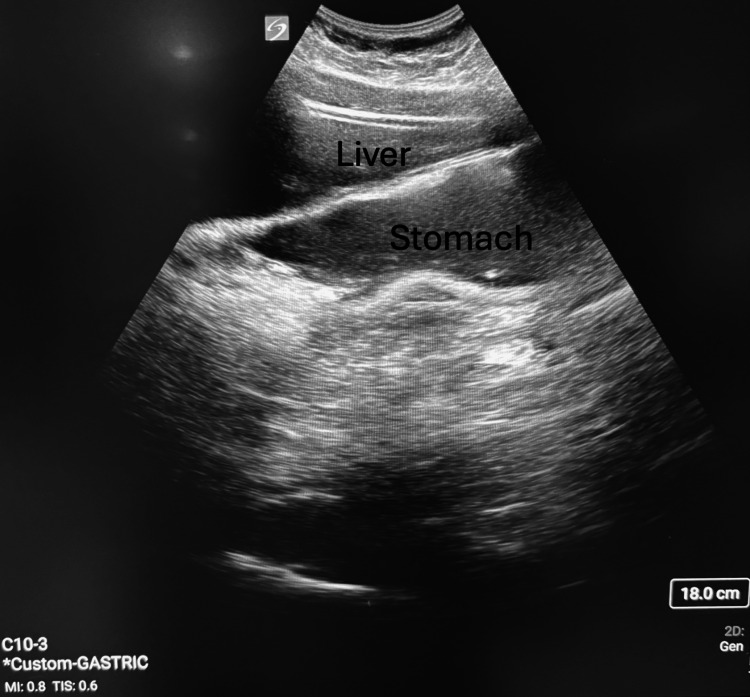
Preoperative gastric PoCUS demonstrating a distended antrum with mixed fluid and solid particulate matter. Stomach labeled with visible gastric antrum; note thick, hyperechoic layering consistent with particulate content. PoCUS: point-of-care ultrasound
